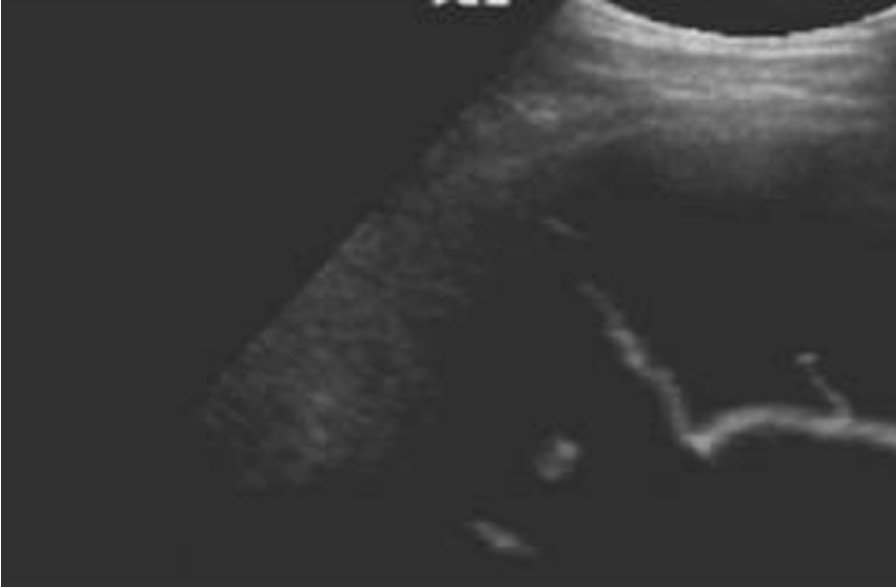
Lung ultrasound image showing a pleural effusion with fibrin stranding in a patient with TB
